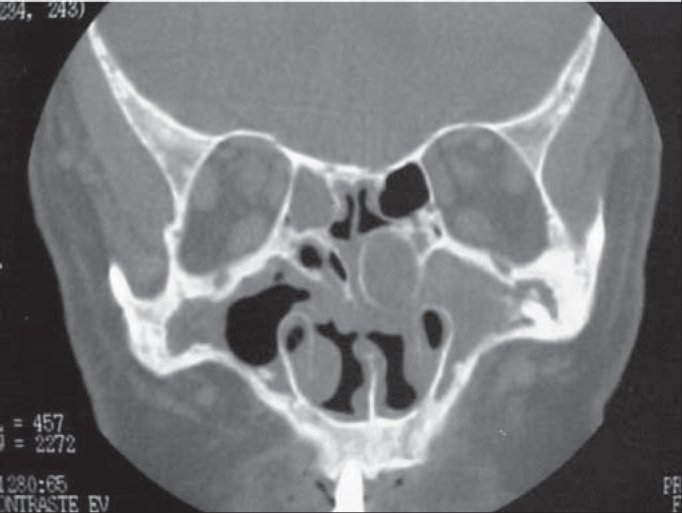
Paranasal sinuses CT scan (coronal section) showing mucopyocele located in the left middle concha.
